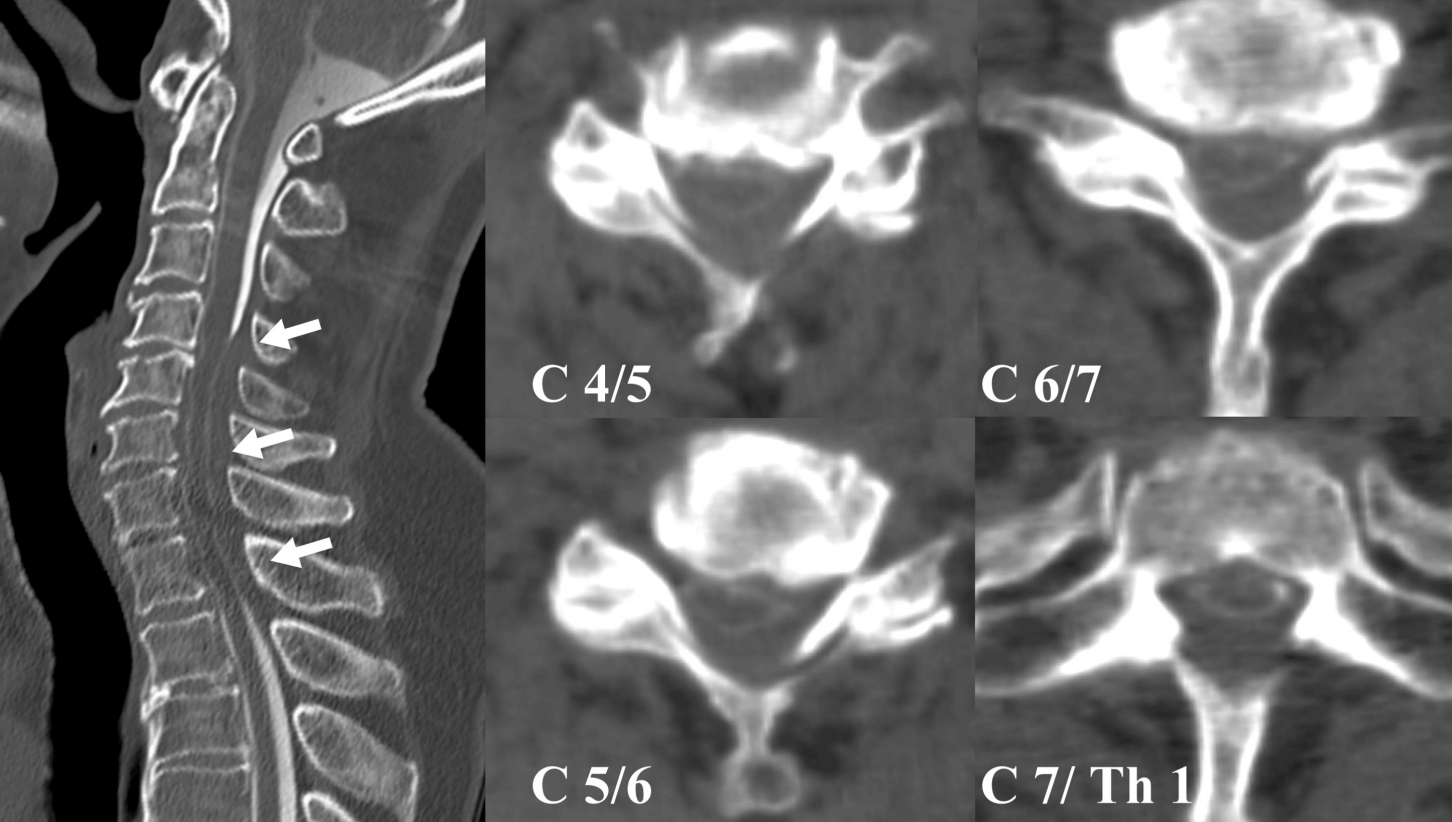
Sagittal and axial images of the myelography CT The defect of contrast enhancement at the C4-Th1 level can be observed, which indicates the cerebrospinal fluid (CSF) blockade (white arrows).
